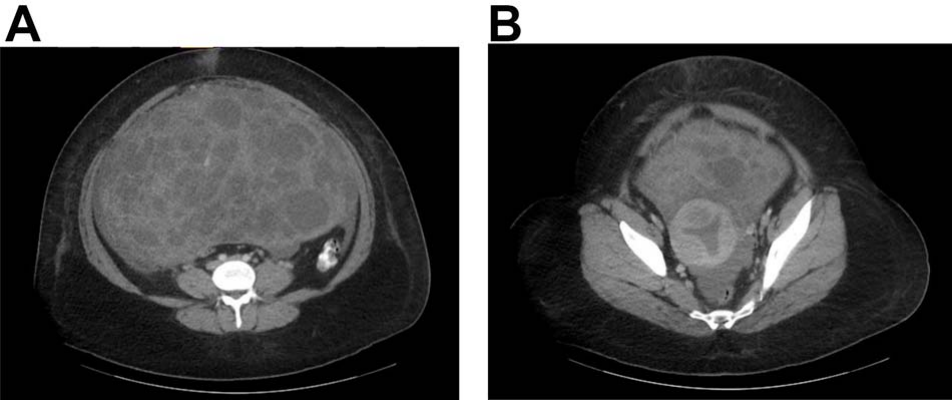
(A) CT abdomen and pelvis with contrast identified a large pelviabdominal mass with internal vascularity measuring 29 × 19 cm and significant ascites; the mass contained both cystic and solid components. (B) The mass encasing the uterus.
